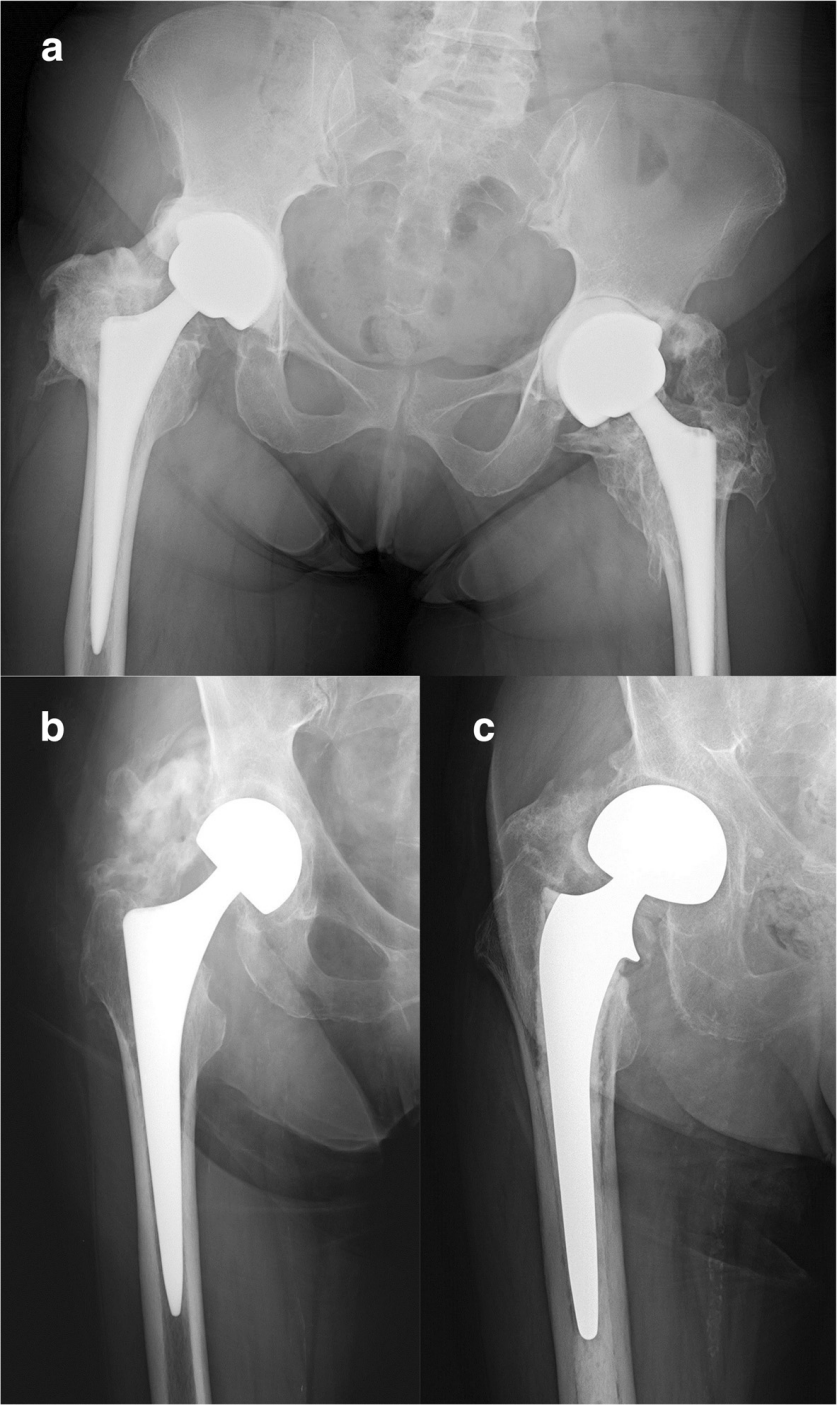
Examples of radiographic high-graded heterotopic ossification for each kind of implant within our cohort. a Ceramic-ceramic-coupled total hip replacement. b TriboFit^®^. c Endoprosthesis
